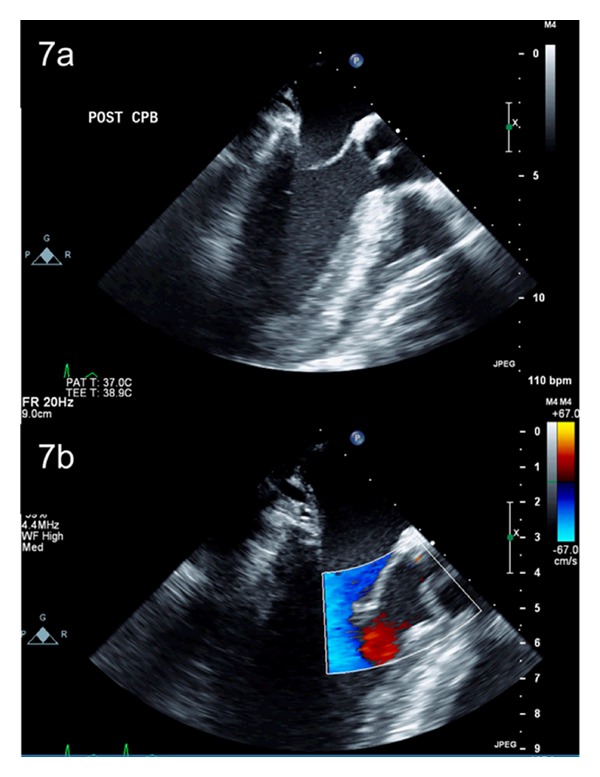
Postoperative transesophageal echocardiogram demonstrating good coaptation of the repaired aortic valve (a), without any residual aortic insufficiency on colour flow Doppler (b).
